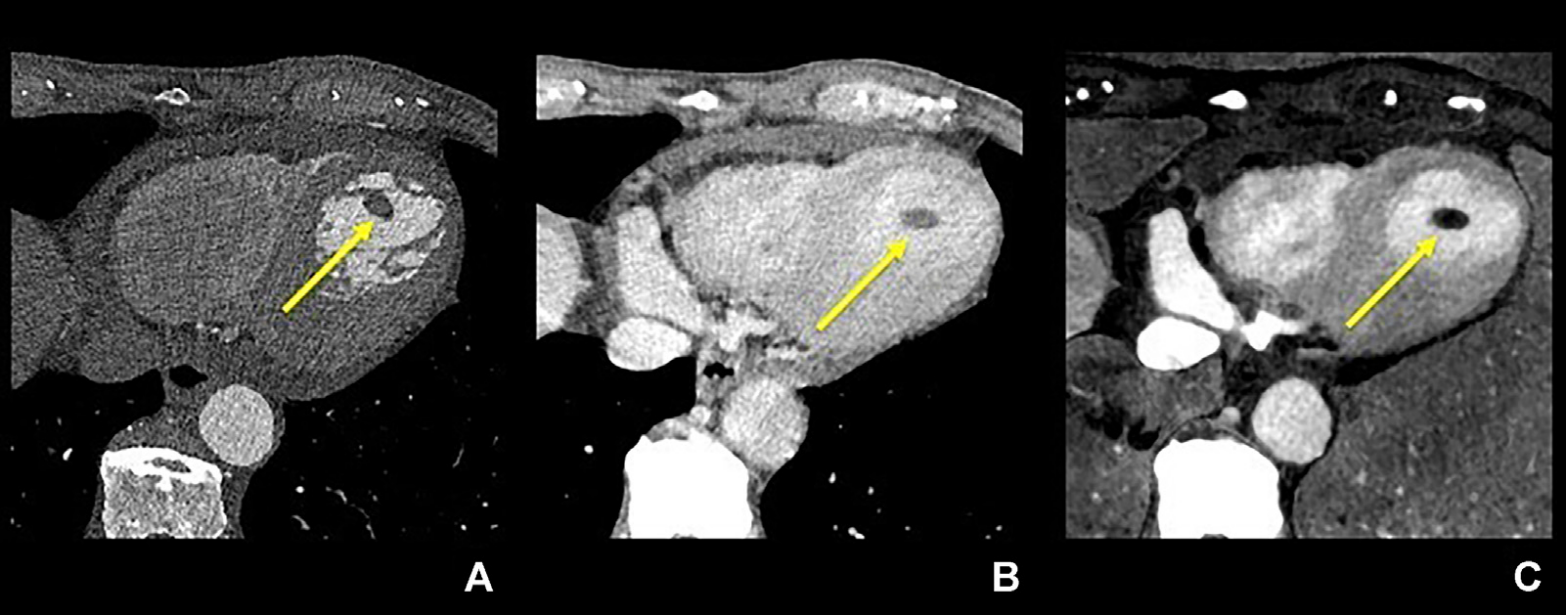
The larger thrombus is visualized on axial images scanned with photon-counting-detector CT: the coronary arterial phase (A), the delayed phase scanned 50 sec after a contrast media injection (B), and the iodine map made from the delayed phase (C) Note that the iodine map better visualizes the thrombus than other 2 images because of the higher contrast between the thrombus and myocardium.
